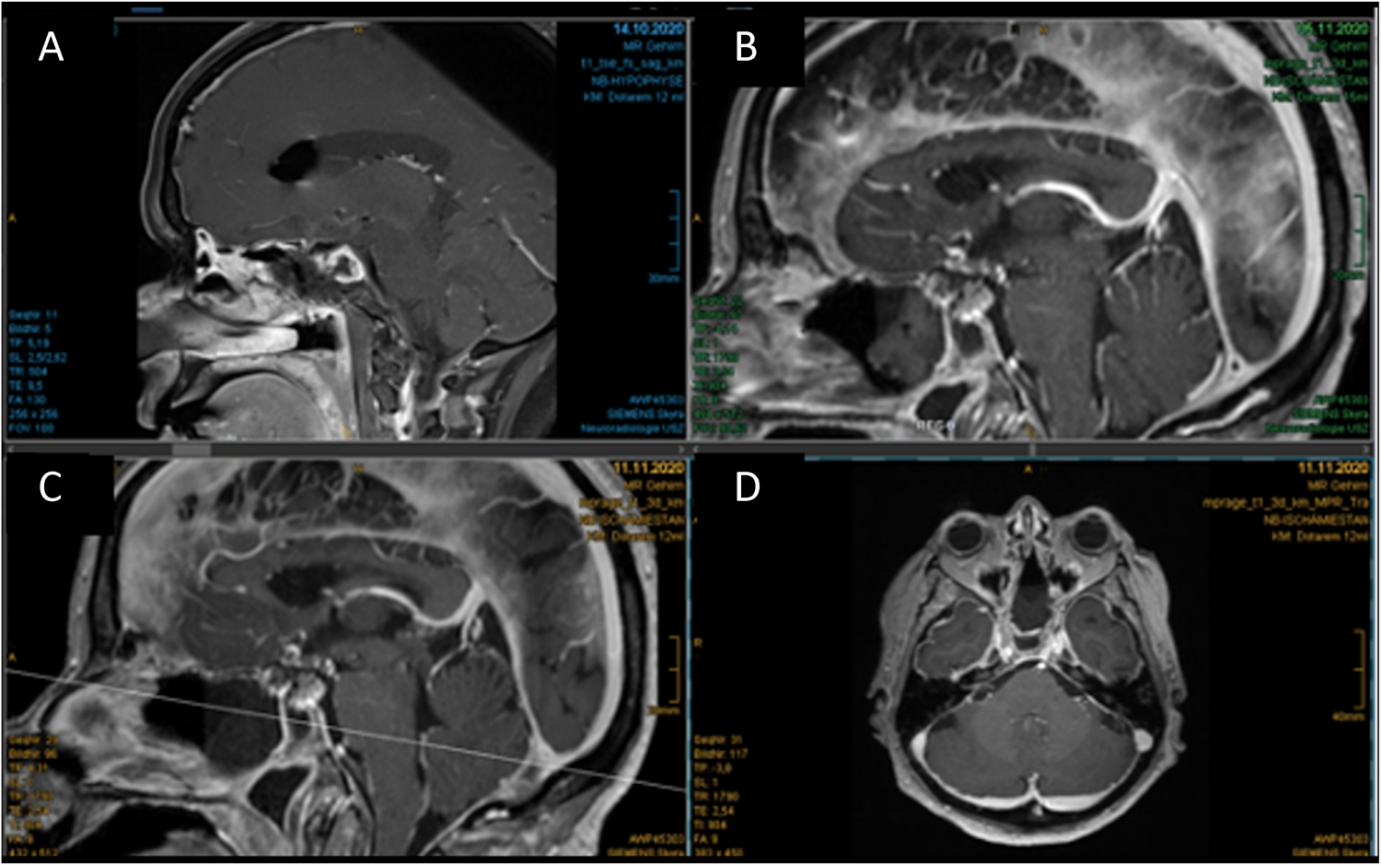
MRI follow-up patient 2 A 16 days before 3rd surgery, B day 6 after 3rd surgery, C day 12 postoperatively (sagittal), and D day 12 postoperatively (transversal) showing no swelling of the patch or signs of infection
